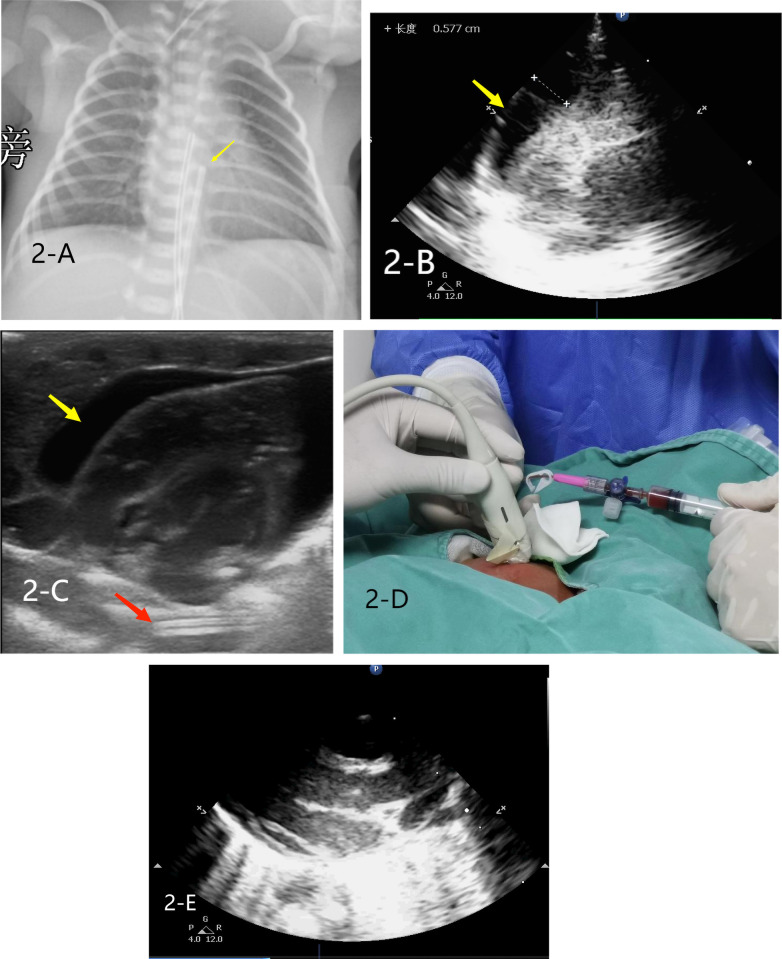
Cardiac POCUS in pericardial tamponade. (A) The tip of the umbilical vein is located at the superior border of T7. (B,C) Apical view showing pericardial effusion (yellow arrow), with the umbilical arterial catheter tip clearly visible in the descending aorta (red arrow) and the UVC tip poorly visualized. (D) Ultrasound-guided pericardiocentesis. (E) Post-drainage resolution of effusion.
